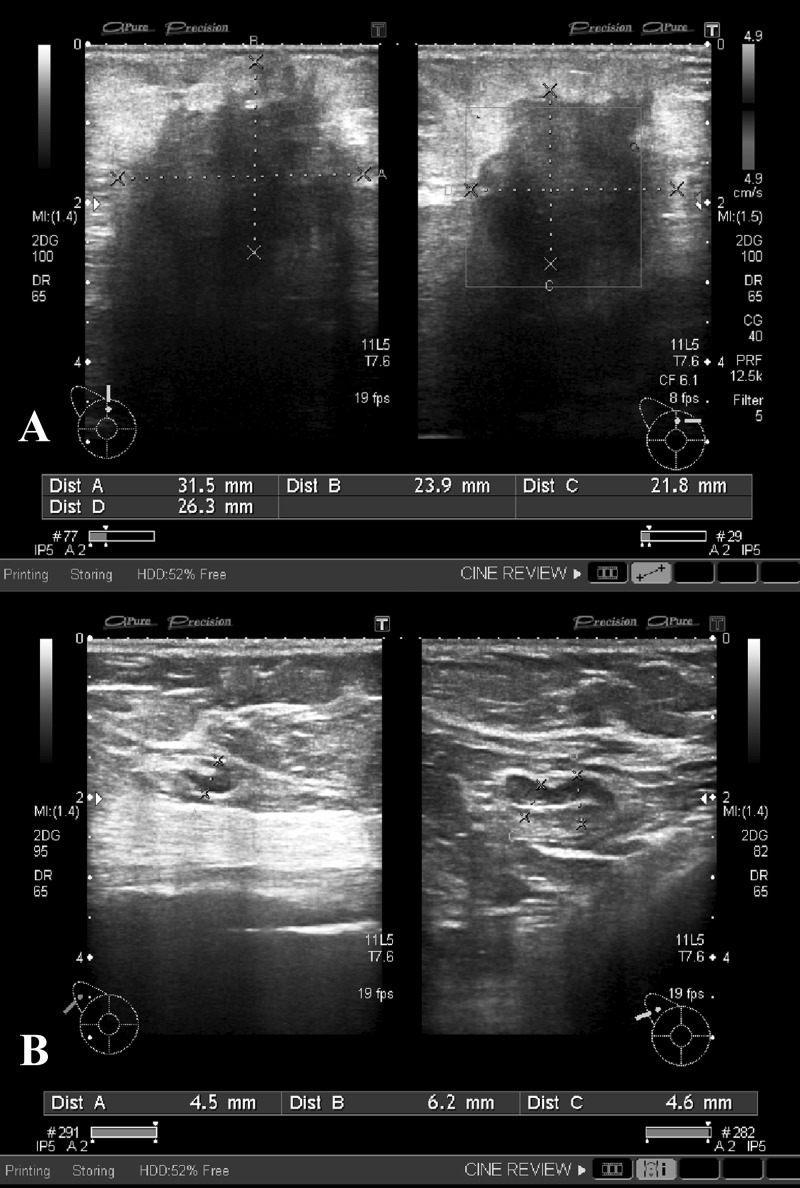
Ultrasound images of right breast and axilla of the patient A) The suspicious neoplastic lesion is redemonstrated in the upper outer quadrant of right breast. B) Subcentimeter lymph nodes with intact fatty hila were identified in the right axilla with thin cortcies and were therefore considered negative for infiltration.
